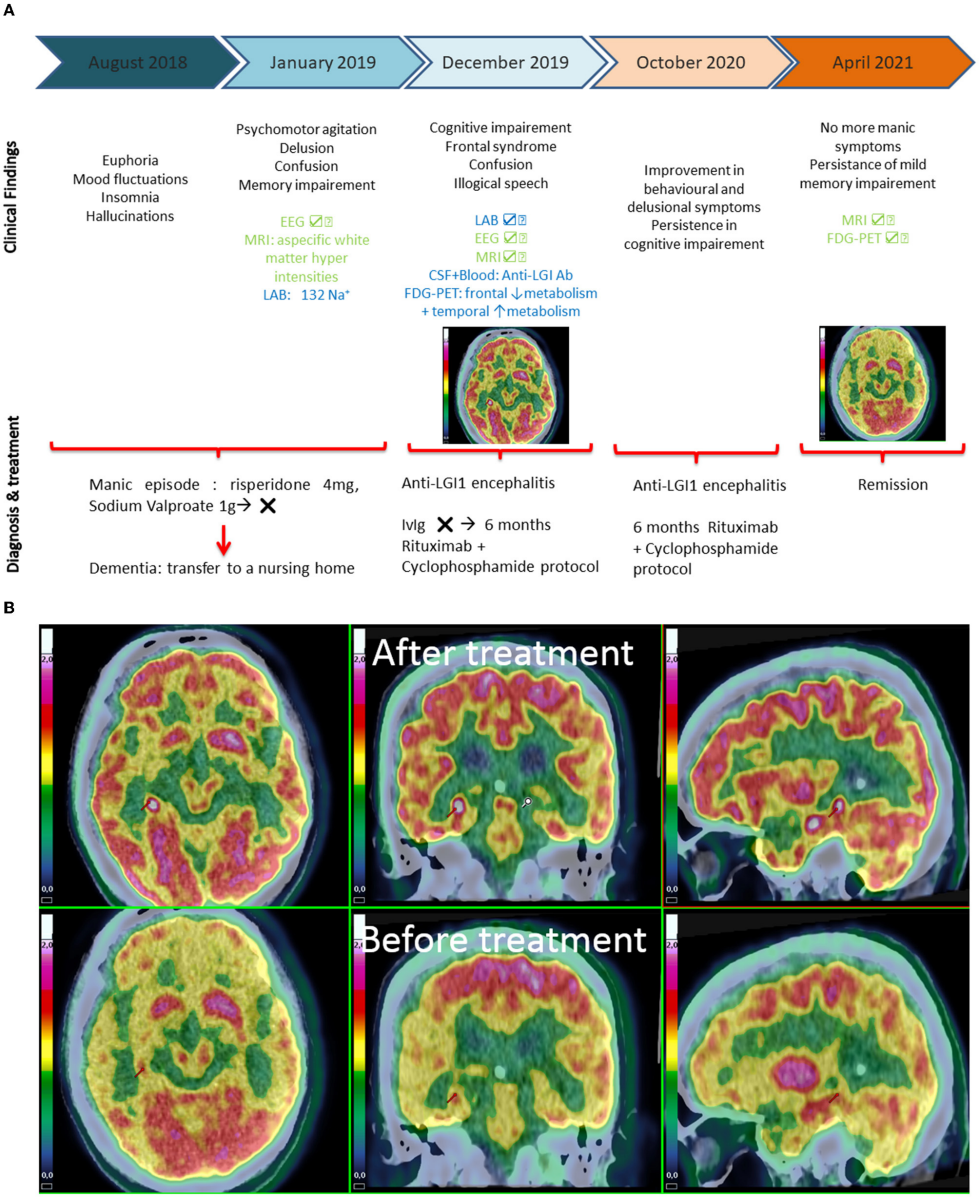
(A) Timeline from first symptoms to diagnosis and final treatment. (B) Brain FDG-PET: axial, coronal and sagittal orientation. Before treatment: diffuse frontal and temporal hypometabolism and striatum hypermetabolism. After treatment: improvement of orbito-frontal lobe and temporal hypometabolism and, to a smaller extent, of dorsolateral lobe hypometabolism. Anatomical orientation (right hemisphere on the right part of the figure). SUV ranging from 0 (blue) to 2 (red).
